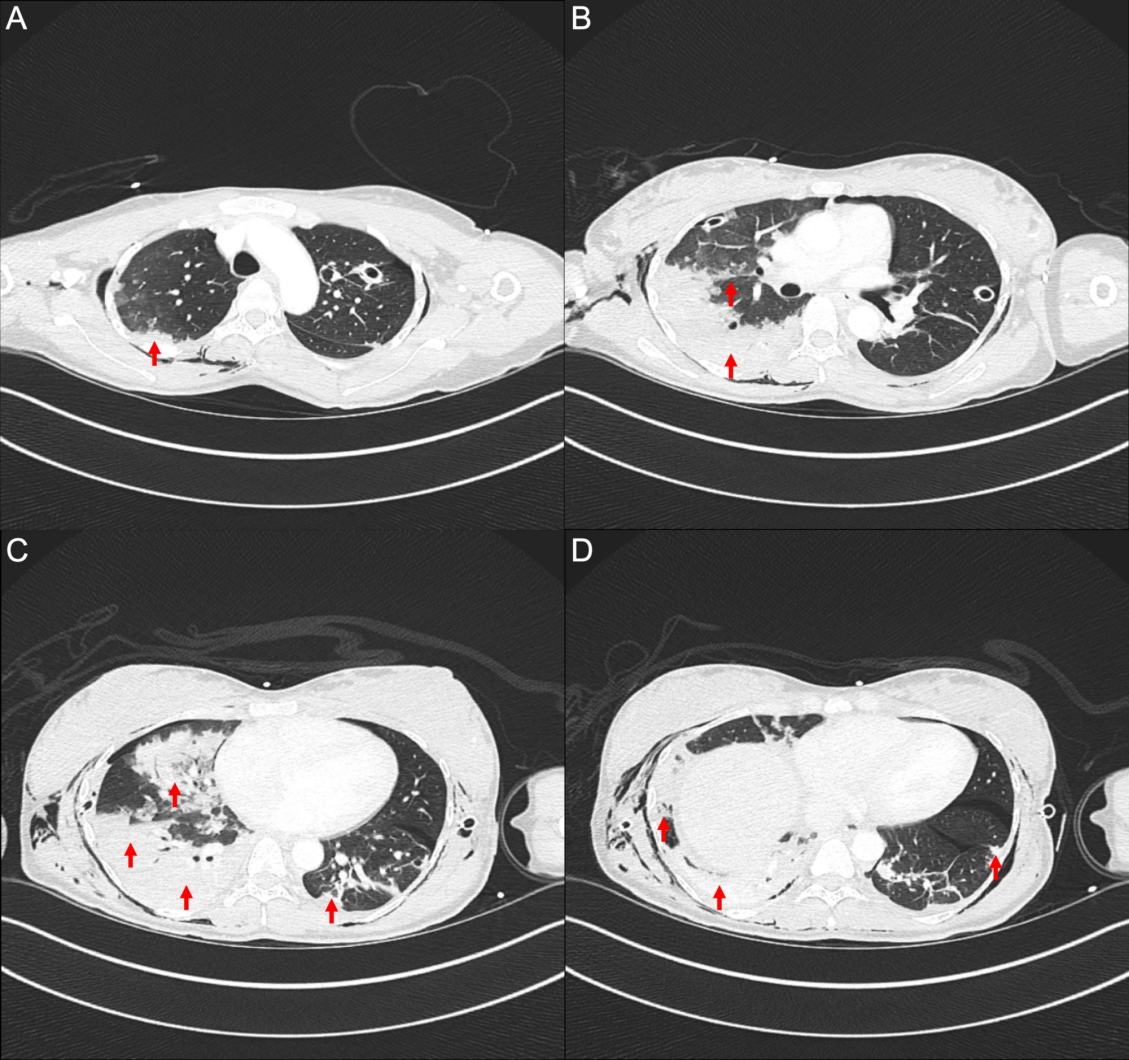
Computed tomography of the chest with iodinated contrast. Selected axial slices from the apices (A) to lung bases (D) demonstrating extensive, predominantly peripheral consolidations and surrounding ground-glass opacities with a crazy paving pattern present throughout the right lung and left lower lobe.
